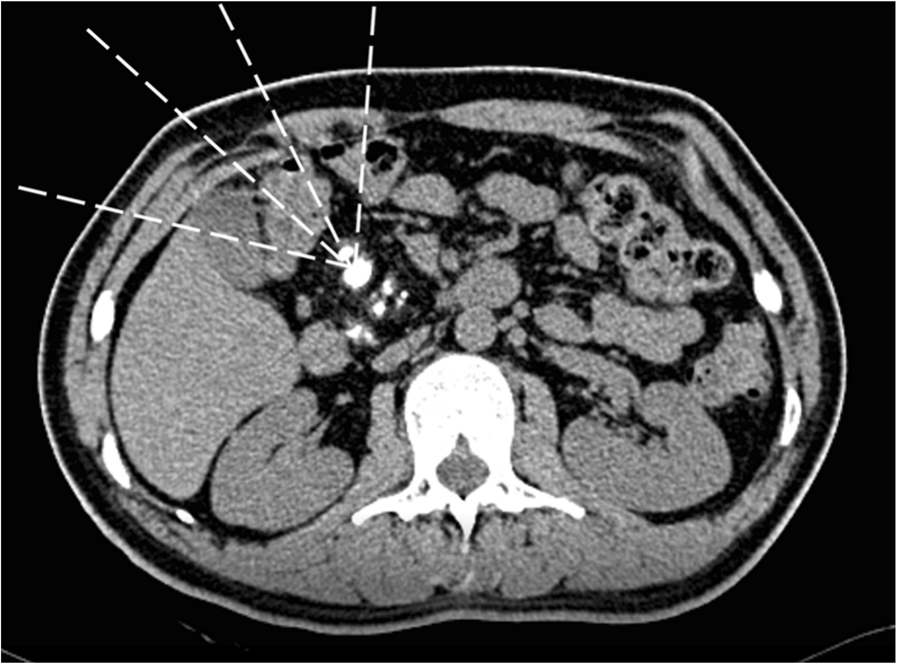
The computerized tomography (CT) shows plenty of radiopaque pancreatic stones in the pancreas. The white dashed lines indicate the shock wave. The conduction pathway of shock wave is a three-dimensional cone space and the hepatic flexure of colon is on the conduction pathway
